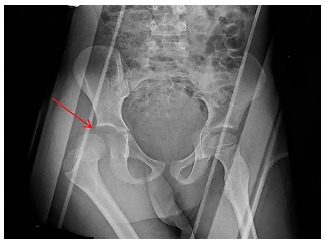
Anterioposterior pelvic radiograph demonstrating superior and lateral displacement of the right femoral head relative to the acetabulum (arrow) and internal hip rotation consistent with posterior hip dislocation.
